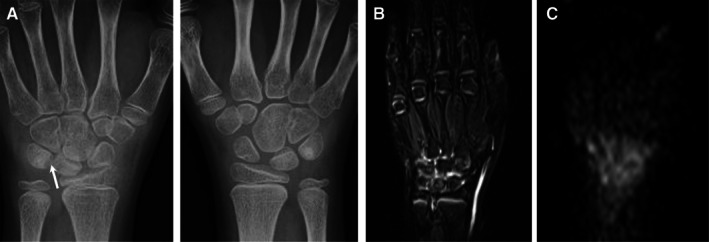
A 7‐year‐old girl with RF‐positive JIA. (A) Radiograph of both hands showed diffuse osteopenia, intercarpal joint space narrowing, and subchondral bone erosion of the lunate‐triquetrum joint of the left side (arrow), which is apparent compared with the less affected right side. (B) Fat‐suppressed T2‐weighted MRI showed intercarpal synovial thickening and increased signal intensities in the carpal bones. (C) Diffusion‐weighted image showed high signal intensity in the intercarpal joints.
